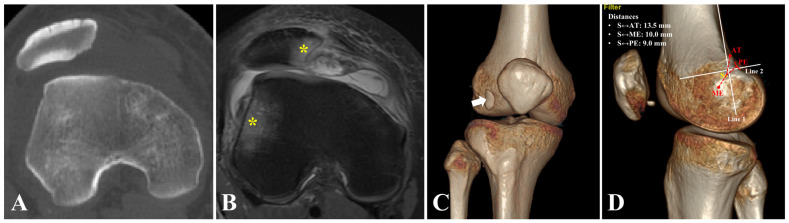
Preoperative imaging evaluation. Right knee. (A) Axial CT scan showing lateral patellar translation. (B) Magnetic resonance imaging showing kissing contusion of the medial patella and the lateral femoral condyle (yellow asterisks). (C) Anterior view of three-dimensional CT reconstruction showing an intra-articular loose body (arrow). (D) Medial view of three-dimensional CT reconstruction showing measurements between the ideal femoral attachment of the MPFL and three anatomic landmarks. The ideal position is assessed with Schöttle’s point (yellow spot). Line 1 is the extension of the posterior cortical line. Line 2 is the line perpendicular to line 1 intersecting the posterior origin of the medial femoral condyle. Schöttle’s point is located 1.3 mm anterior to line 1 and 2.5 mm distal to line 2. Preoperative measurements demonstrate that the ideal femoral tunnel lies 13.5 mm to the AT, 10.0 mm to the ME and 9.0 mm to the PE. AT, adductor tubercle. CT, computed tomography. ME, medial epicondyle. PE, posterior edge. S, Schöttle’s point.
